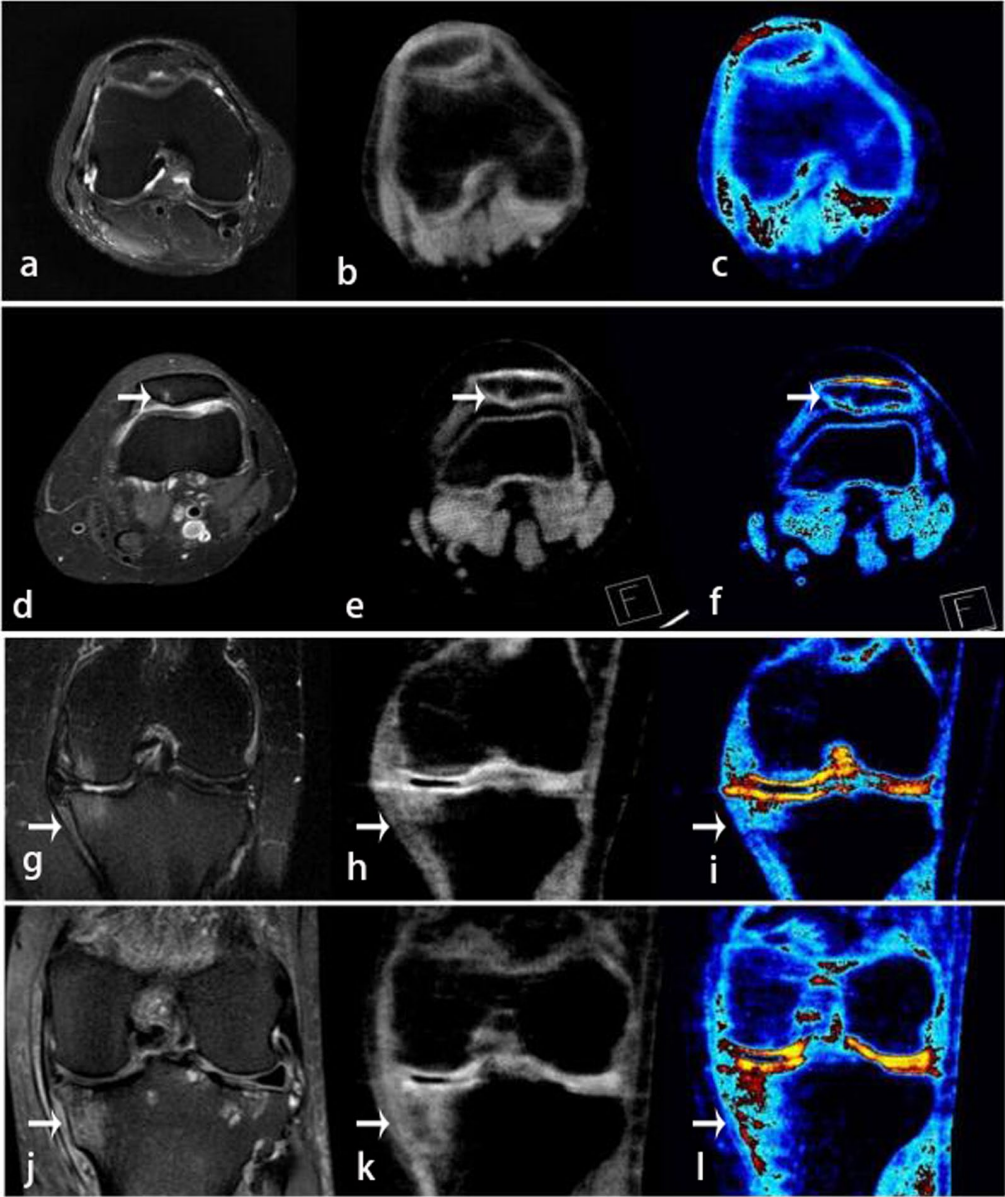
a–c Axial MRI, DECT VNCa, and color-coded map images showing Grade 1 ELMSI in the patella. d–f Axial MRI, DECT VNCa, and color-coded map images showing Grade 2 ELMSI in the patella. g–i Coronal MRI, DECT VNCa, and color-coded map images showing Grade 3 ELMSI in the tibial plateau. j–l Coronal MRI, DECT VNCa, and color-coded map images showing Grade 4 ELMSI in the tibial plateau (arrow)
